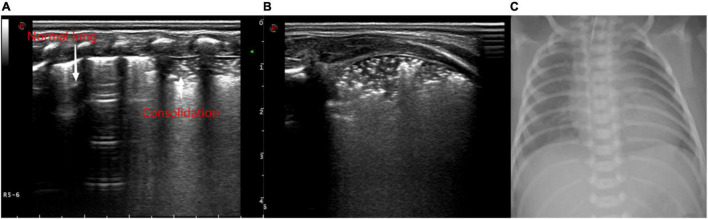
Ultrasonic manifestations of neonatal lung consolidation. Pulmonary ultrasound shows an irregular consolidation area with a hyperechoic air bronchogram sign. It shows that dot or linear hyperechoic artifacts vary with breathing in the lung consolidation tissue and pleural lines. Normal lung tissue can be observed at its location. (A) Longitudinal image of intercostals. (B) Transverse image of intercostals. (C) X-ray showing multiple patchy fuzzy shadows in both lungs.
